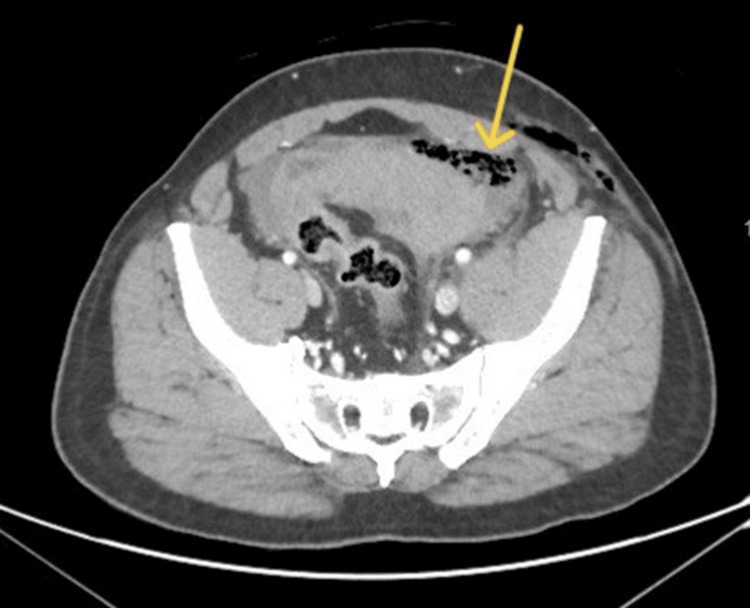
Computed tomography (CT) scan The image shows fecal contamination outside the bowel loops in the left lower abdomen (arrow), suggestive of colonic injury.
